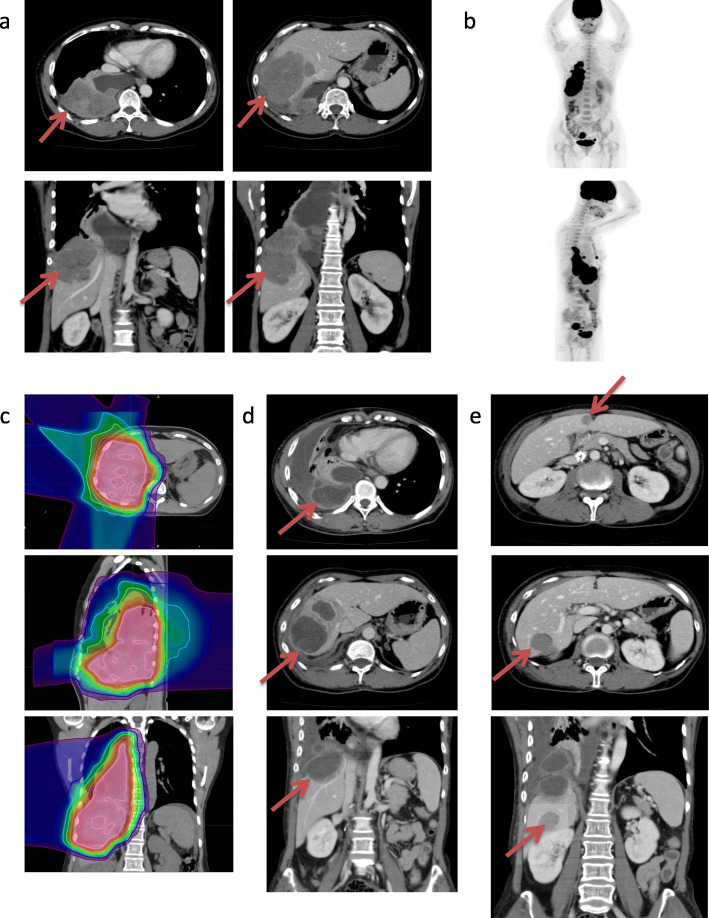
Diagnostic image after recurrence around the right diaphragm, liver, and thoracic cavity. a Enhanced computed tomography (CT) image before proton therapy, and after chemotherapy. Red arrows indicate the tumor between the right diaphragm and liver. b Positron emission tomography-CT image during the same time as in “a”. c Planning of proton therapy. d Enhanced CT after 4 months of proton therapy. The tumors in the irradiation field disappeared and were liquefied. Red arrows indicate the liquefied tumors. e Enhanced CT image when liver metastases increased. Red arrows indicate the liver metastases
